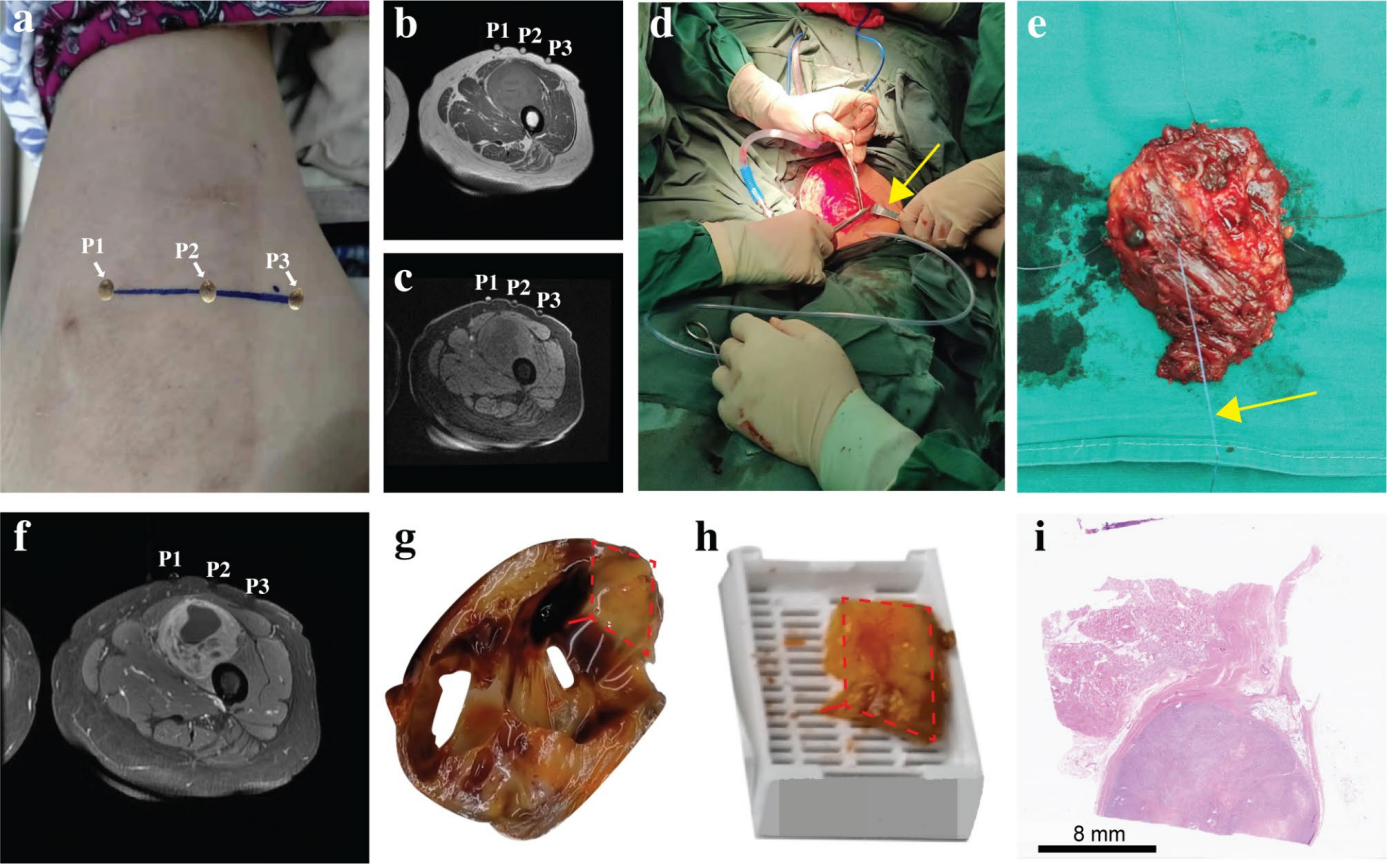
MRI-Pathology control method
